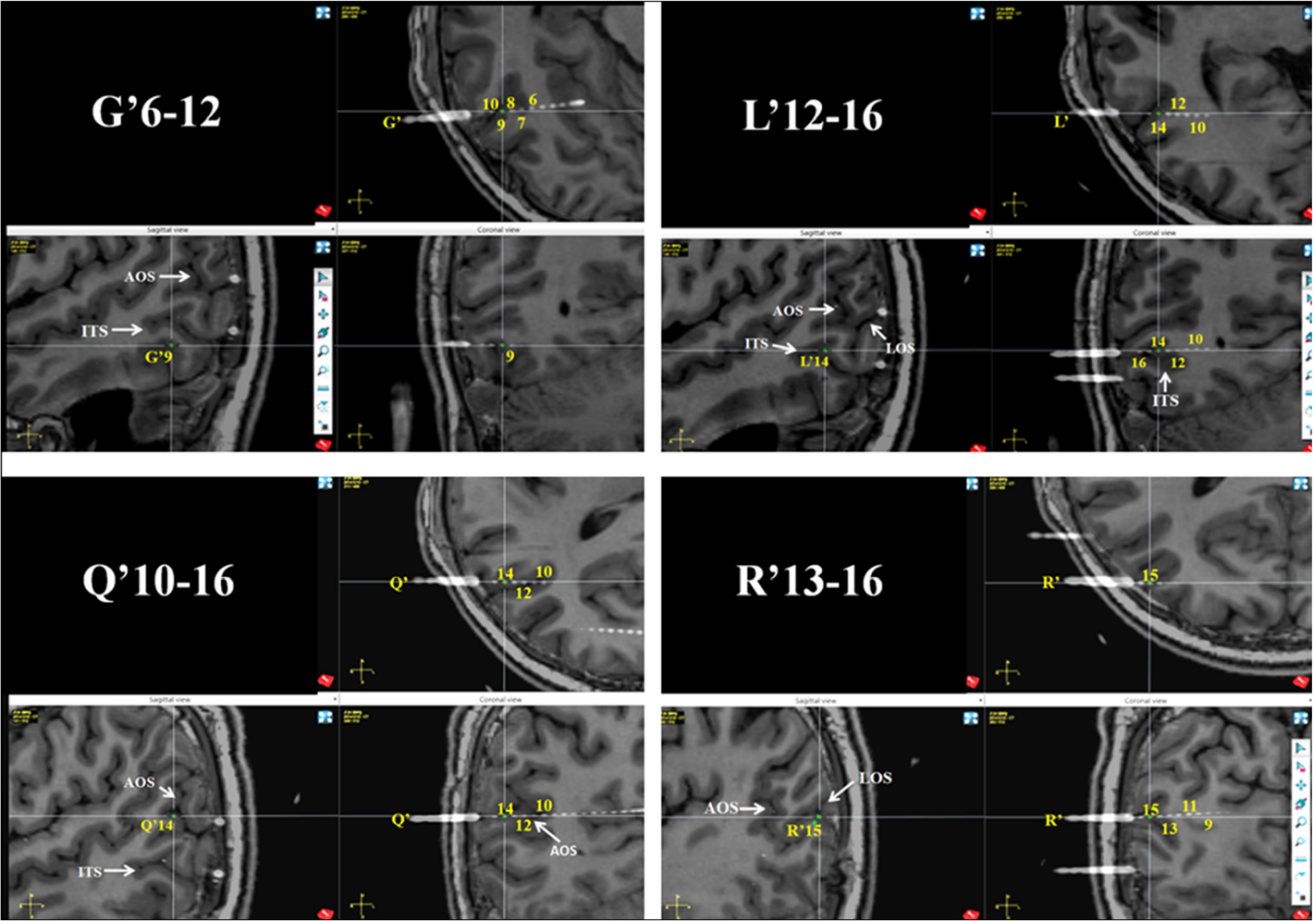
The reconstruction of the exact location of key electrodes through the fusion of postoperative CT and preoperative MRI data. AOS: anterior occipital sulcus; ITS: inferior temporal sulcus; LOS: lateral occipital sulcus
